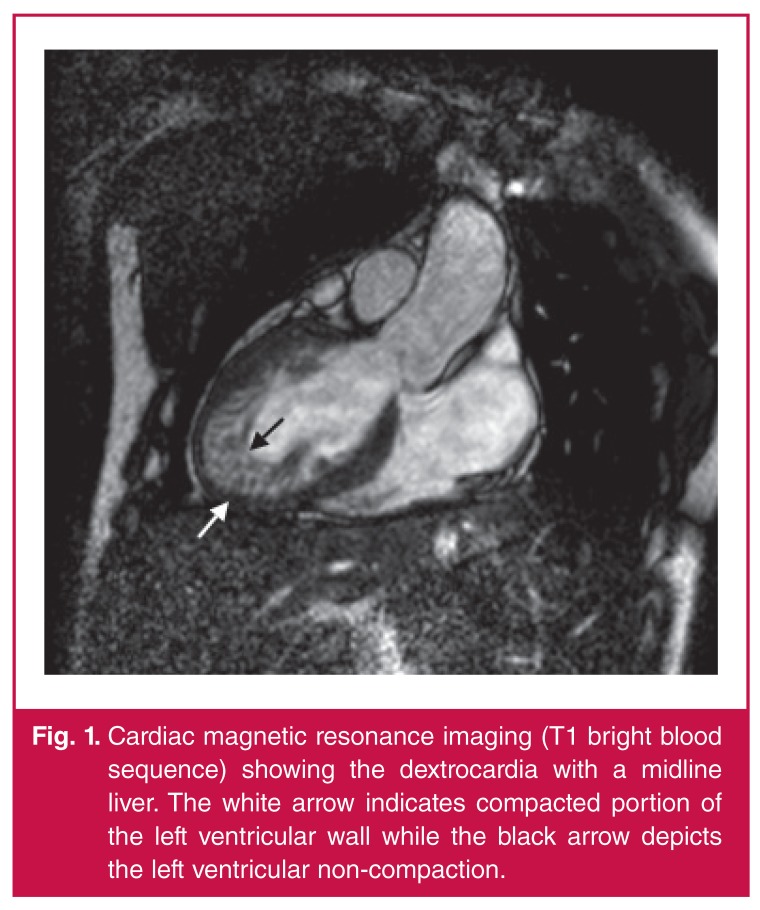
Cardiac magnetic resonance imaging (T1 bright blood sequence) showing the dextrocardia with a midline liver. The white arrow indicates compacted portion of the left ventricular wall while the black arrow depicts the left ventricular non-compaction.
